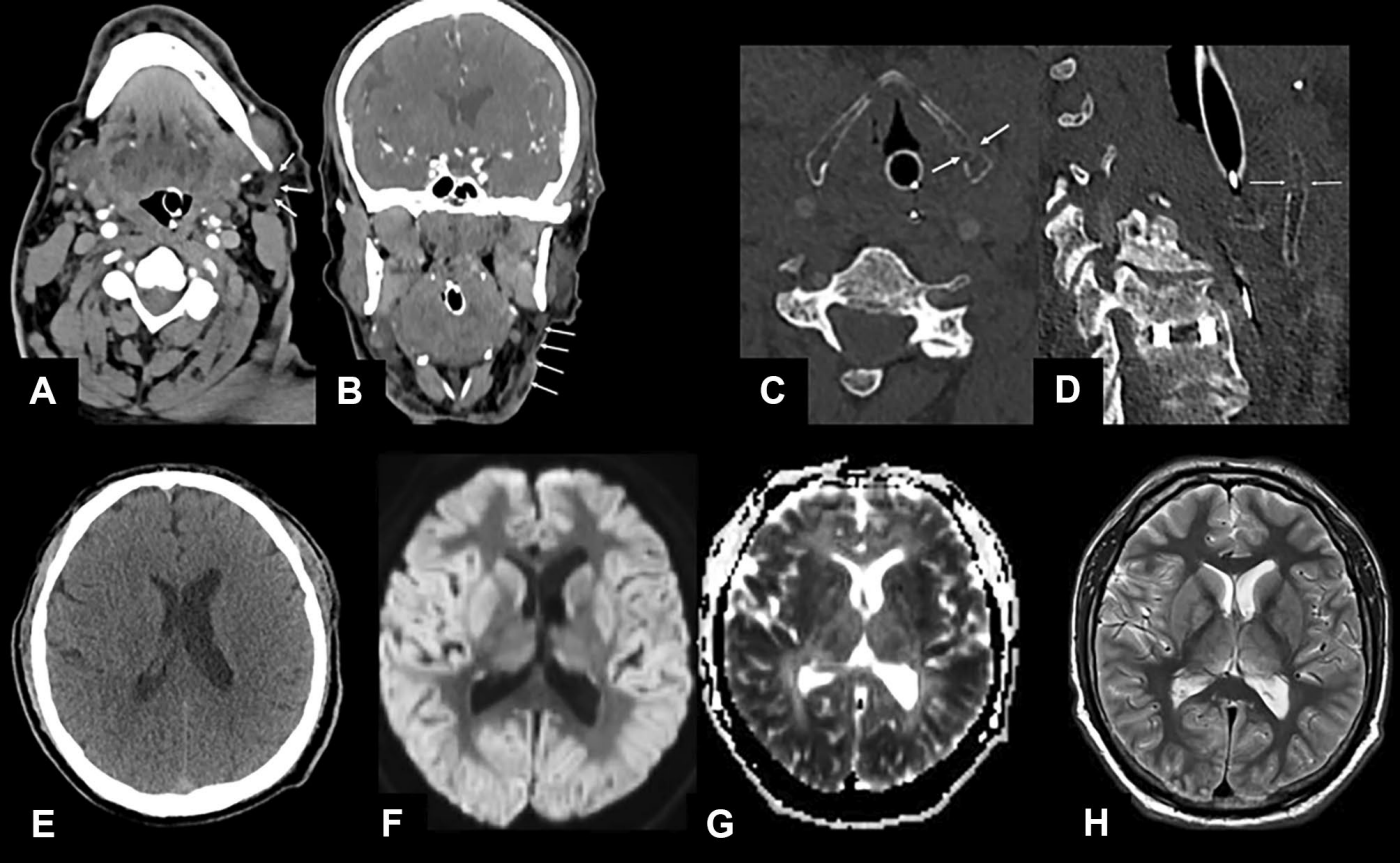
Axial and coronal NECT; soft tissue (3 mm ST) and bone window with a ST of 1 mm; axial and paracoronal slices. Non-enhanced CT and MRI of the brain with DWI (ST 3 mm) and ADC maps and a T2-weighted axial image (ST 3 mm). The axial (A) and coronal (B) NECT images reveal marked edema and stranding of the subcutaneous fat and cutis in the left submandibular region with edematous thickening of the cutis (arrows). The bone window of the larynx brought up a left-sided fracture of the thyroid cartilage with a clear kinking (arrows in C and D). On the non-enhanced emergency CT scan of the brain, diminished corticomedullary differentiation (E) is seen. The MRI scan of the brain (36 h after the initial event) confirmed the severe hypoxic-ischemic encephalopathy with diffusion restriction of the fronto-temporo-parieto-occipital cortex bi-hemispherically symmetric with involvement of the thalami and basal ganglia (F and G) with hyperintense demarcation on the T2-weighted image (H)
